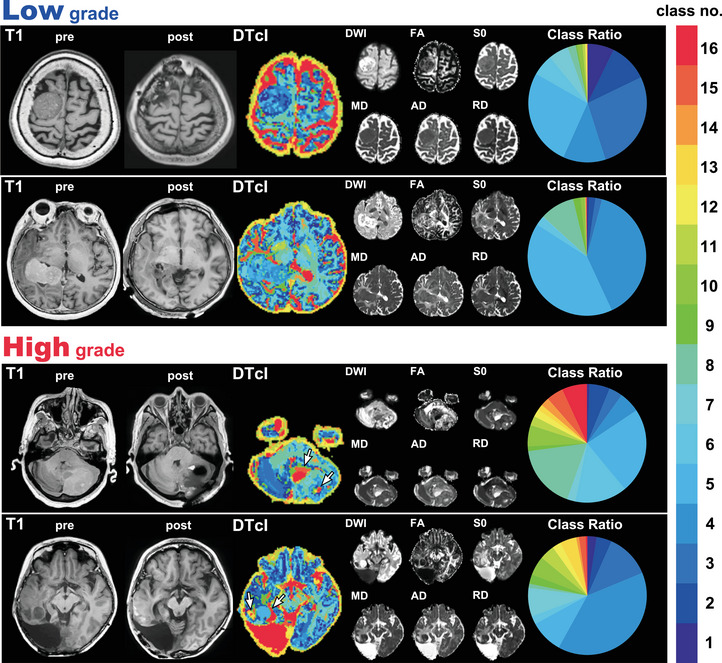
Representative cases of low‐ and high‐grade meningiomas, including the 16‐class DTcIs that showed the highest classification performance. The preoperative and postoperative T1‐weighted images, DTcIs, six diffusion tensor images, and the ratios in each class number are shown for each patient. The arrows show the areas of warm‐colored classes, which indicate WHO high grades. The colors on the DTcIs and circular charts correspond to the class numbers shown in the color bar. DWI, diffusion‐weighted imaging; FA, fractional anisotropy; S0, raw T2 signal without diffusion weighting; MD, mean diffusivity; AD, axial diffusivity; RD, radial diffusivity; DTcI, diffusion tensor‐based clustering image; WHO, World Health Organization.
